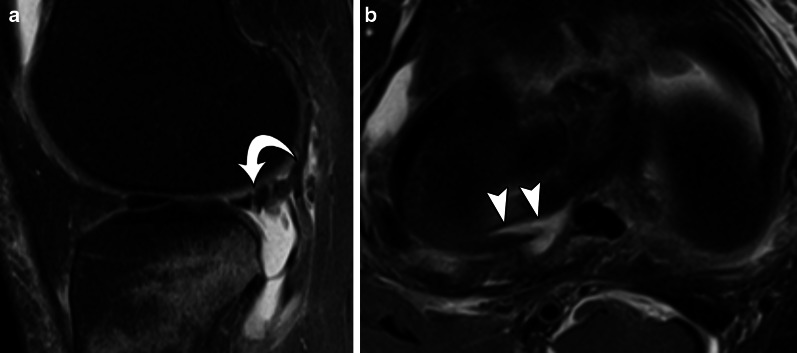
Sagittal T2-weighted fat-suppressed MR image (a) shows a zip lesion, with a rip between *Wrisberg* ligament (curved arrow) and posterior horn of lateral meniscus. Axial T2-weighetd fat-suppressed MR image (b) shows the lesion in a cross-sectional view (arrowheads)
